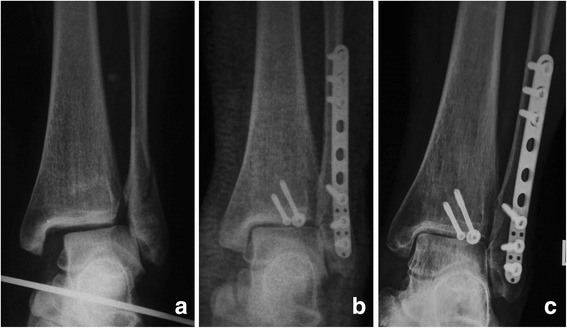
a The preoperative X-ray showed an AO/OTA type-B ankle fracture. b The patient was treated with open reduction and internal fixation of lateral and posterior malleolus, and the medial clear space was back to normal without surgical repair of the deltoid ligament. c Two years followup show good reduction of the medial clear space
